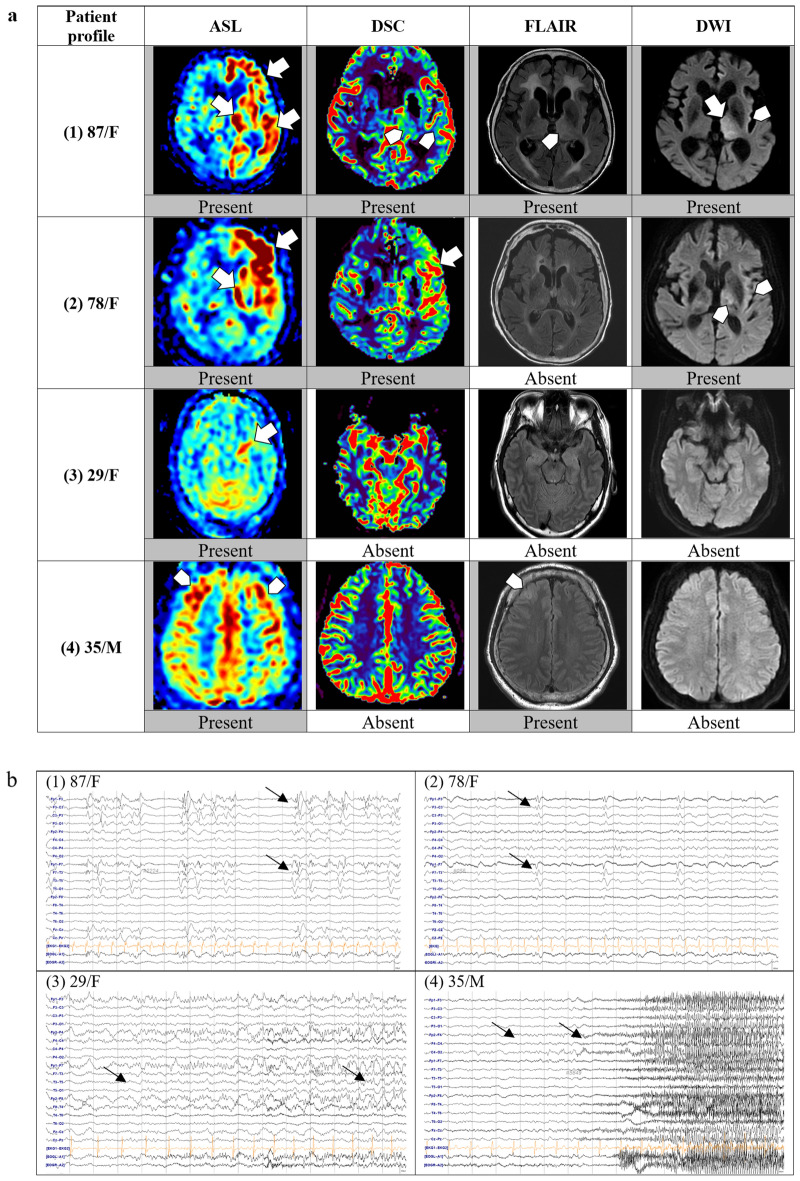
Examples of brain MRI sequences and EEGs of four representative patients with status epilepticus. Brain MRI and EEG of each patient were listed according to the same number from (1) to (4). (a) Brain MRI: (1) ASL showing hyperperfusion in the left frontal and temporal cortices and the left thalamus. DSC reveals subtle increases in perfusion in the left thalamus and lateral temporal lobe. FLAIR and DWI show changes in the left thalamus and insula. (2) ASL and DSC show definite hyperperfusion in the left frontal and temporal lobes, FLAIR shows no changes, and DWI shows subtle restrictions in the left thalamus and insula. (3) ASL shows definite hyperperfusion in the left hippocampus, but no signal changes are noted on DSC, FLAIR, or DWI. (4) ASL showing subtle hyperperfusion in the bilateral frontal cortices, and subtle T2 hyperintensity is noted in the left frontal cortex. (b) EEG: (1) Left frontotemporal periodic discharges. (2) Left frontotemporal periodic discharges. (3) Left temporal rhythmic delta activity with evolution indicating an electrographic seizure. (4) Right frontal theta rhythm evolving to beta activity, indicating an electrographic seizure. *ASL* arterial spin labeling; DSC, dynamic susceptibility contrast, *DWI* diffusion-weighted imaging, *EEG* electroencephalogram, *FLAIR* fluid-attenuated inversion recovery, *MRI* magnetic resonance imaging.
